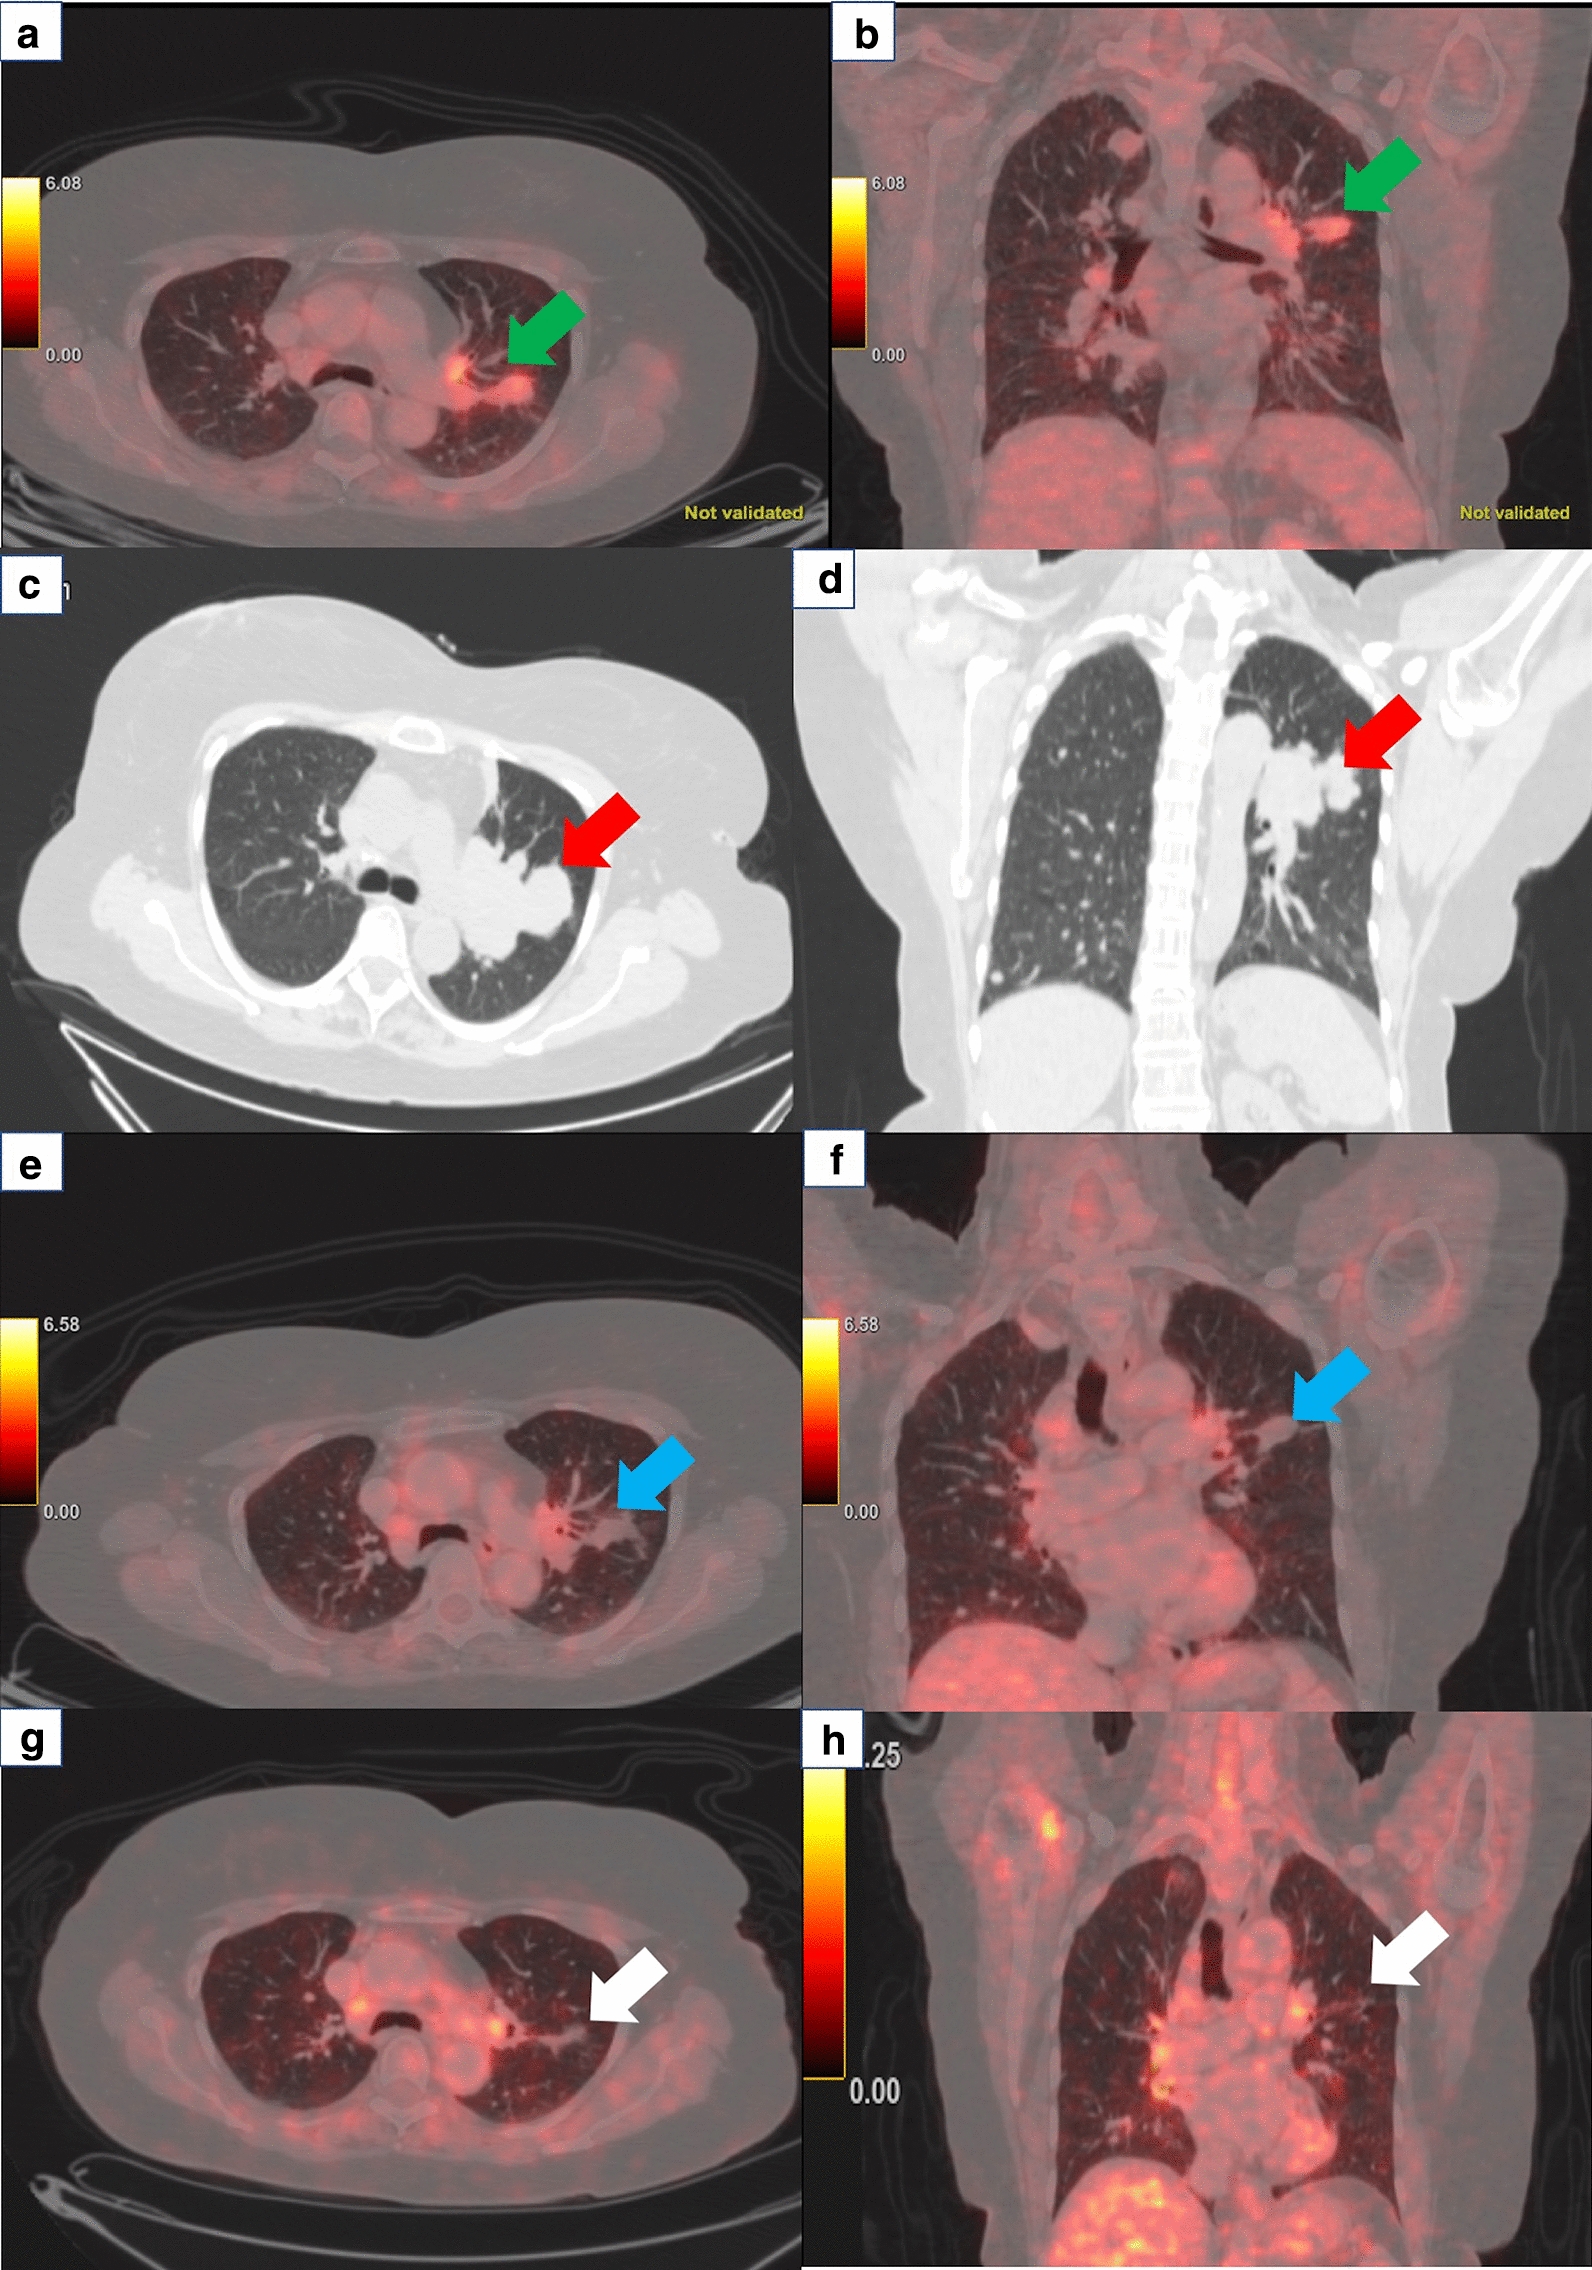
Axial (a) and coronal (b) fused positron emission tomography—computed tomography (PET-CT) images showing 18-fluorodeoxyglucose (18-FDG) avid soft tissue nodule in the left lung upper lobe (green arrows) after completion of gemcitabine-docetaxel chemotherapy. CT scan images (c, d) in lung window showing progression of nodules in left lung upper lobe (red arrows) after 3 months of watchful waiting. 18-FDG PET-CT scan images showing reduction in size with resolution of metabolic activity of lung nodules after 3 months (e, f) (blue arrows) and 9 months (g, h) (white arrows) of treatment with pembrolizumab + pazopanib
